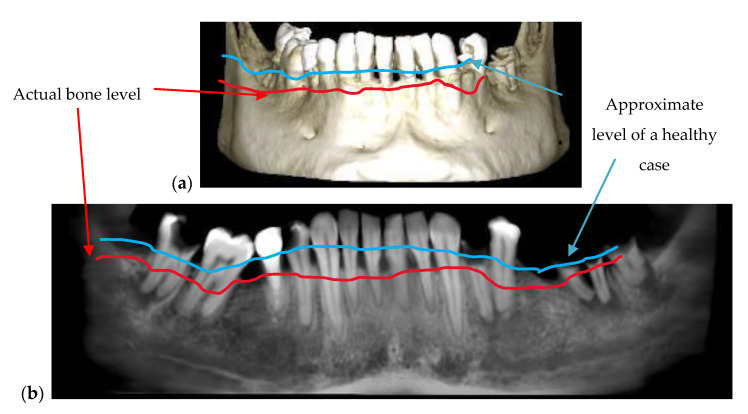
Periodontitis disease observed on a 3D CBCT reconstruction (a) and on a panoramic radiography (b). Patient C.O., male, age 34 years, diagnosed with periodontitis, alongside other dental issues such as cavities and dental abscesses.
